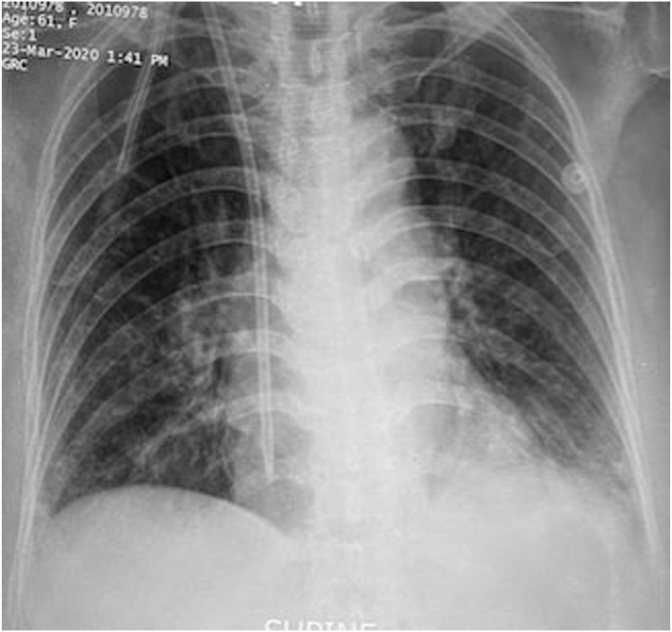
Plain chest X-ray revealed consolidation in the basal lung sinistra.
